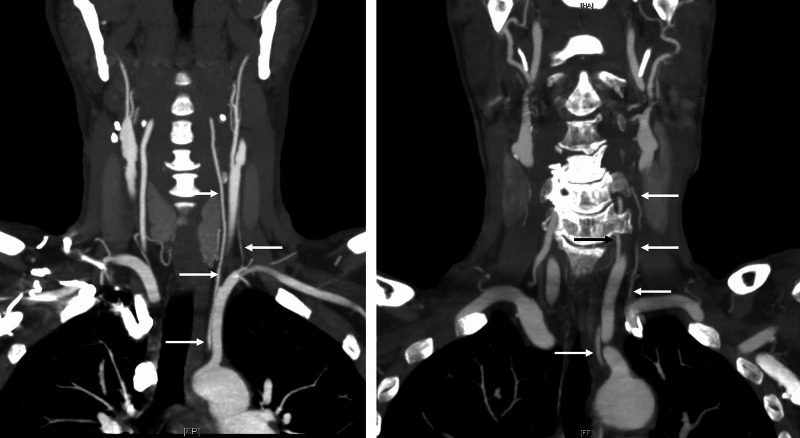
Coronal CTA of two patients with accessory vertebral arteries (right arrows) Both patients also have an aortic arch origin of the left vertebral artery (left arrows). CTA: CT Angiogram
